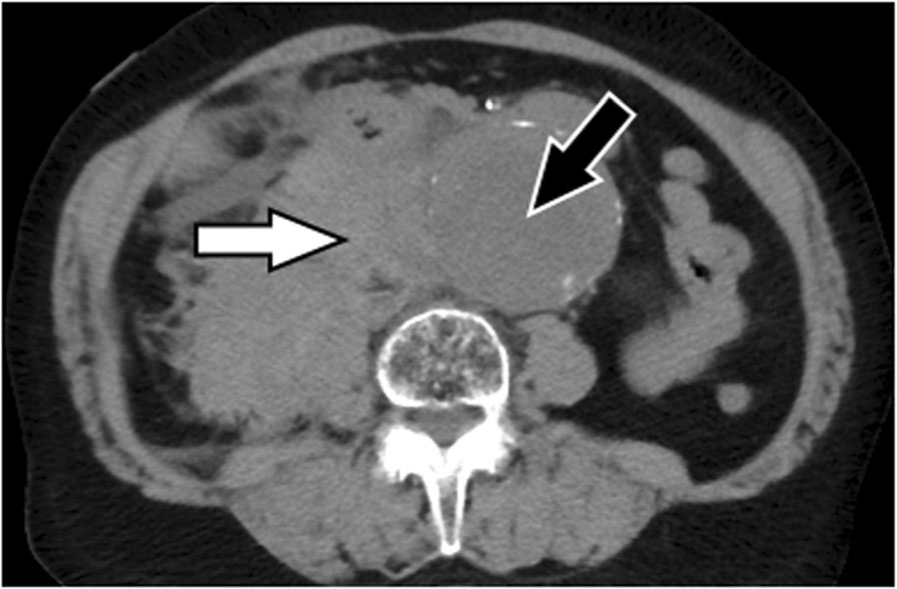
Noncontrast computed tomography of the abdominal aortic aneurysm (black arrow) with an adjacent subacute retroperitoneal hemorrhage (white arrow)
